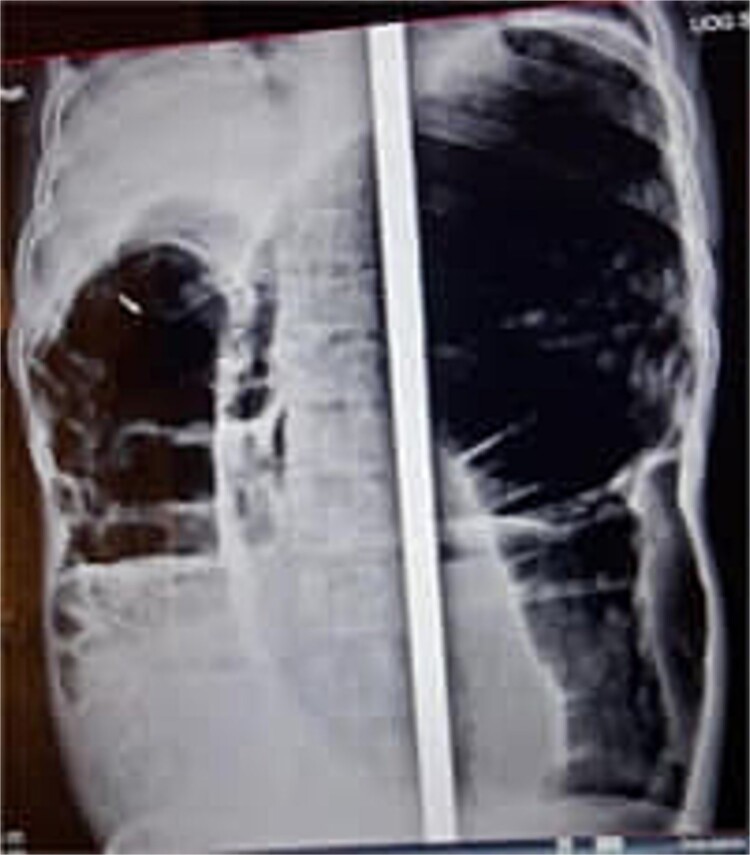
Abdominal X-ray showing dilated colon and multiple air-fluid levels.
